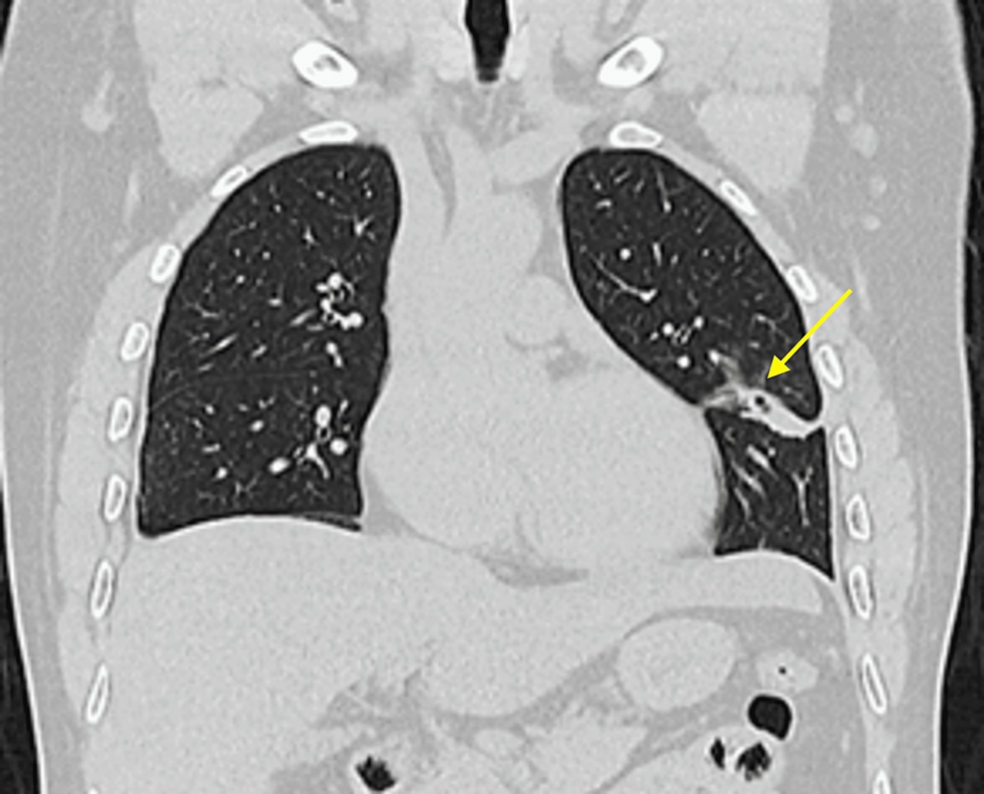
Repeat lung CT showed a decrease in the size of the cavitated subpleural lesion at seven days of treatment CT: computed tomography
